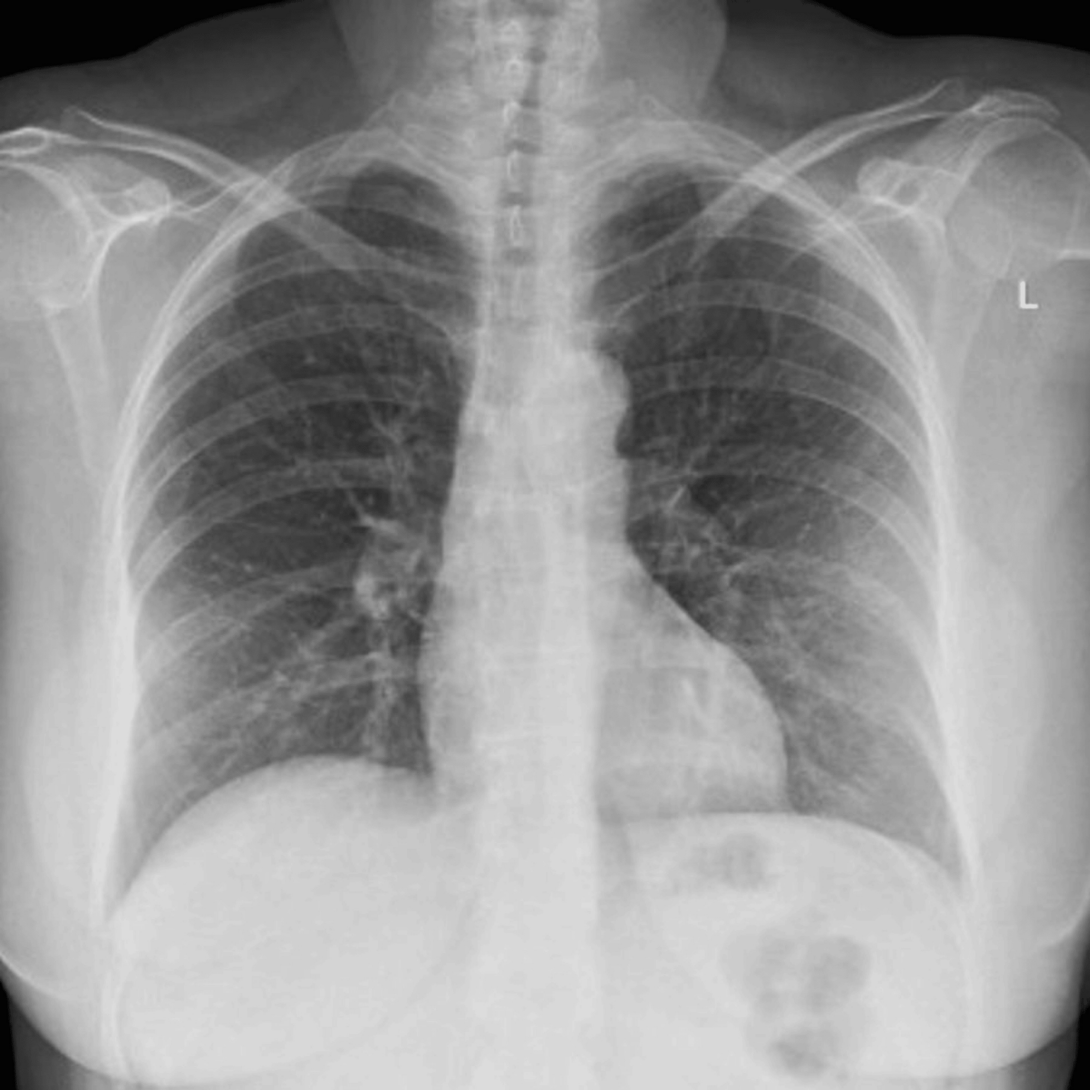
Chest X-ray one day prior to admission.
